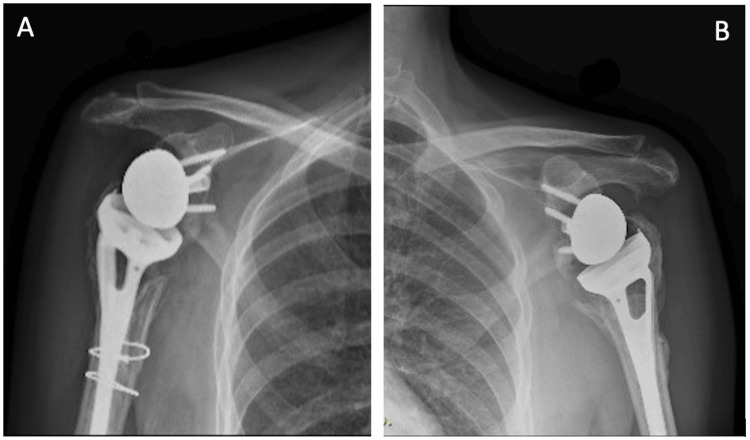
X-ray anteroposterior view of the right (A) and left (B) shoulders (8 months post-operative) shows no signs of loosening or scapular notching.
